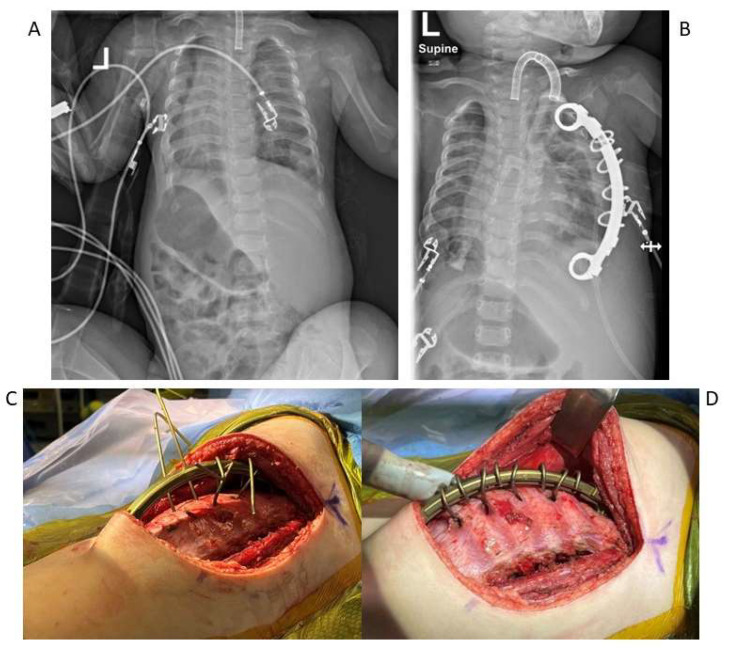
(A,B) Spine and chest radiographs of a patient with Jeune syndrome showing a preoperative constricted chest cavity and postoperative appearance post staged expanding thoracoplasties with insertion of bilateral rib-to-rib VEPTRs. (C,D) Intraoperative photos of expanding thoracoplasties with rib-to-rib VEPTR insertion.
